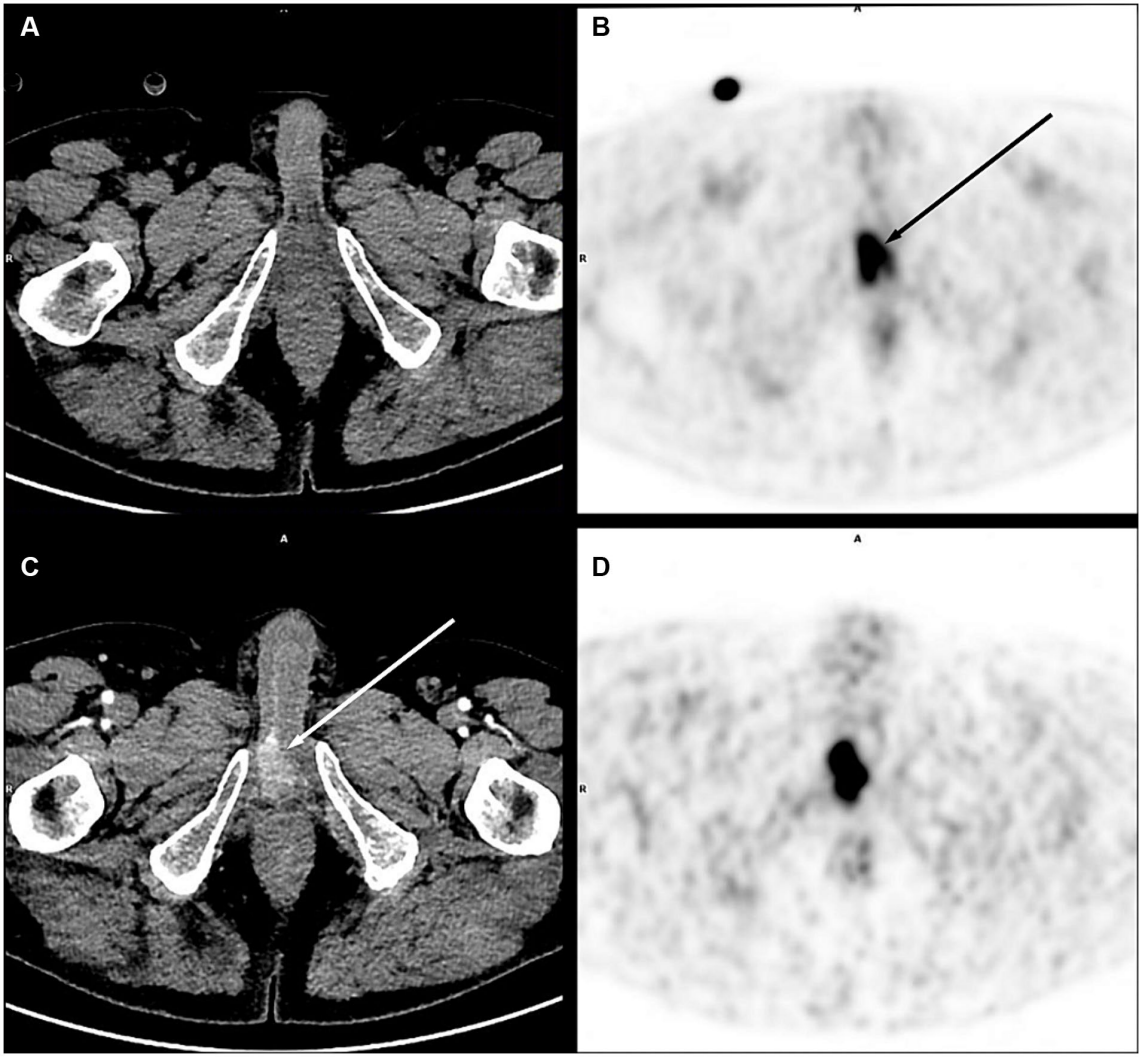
Right corpora cavernosa metastasis from penile carcinoma. on PET images (early acquisition B, late acquisition D), there was a focal uptake [(B) black arrow], without significant lesion on unenhanced CT (A), in an area close to the physiological urinary activity. With contrast medium injection on a dedicated acquisition (C), this uptake corresponded to a metastatic lesion clearly visible on CT (white arrow).
